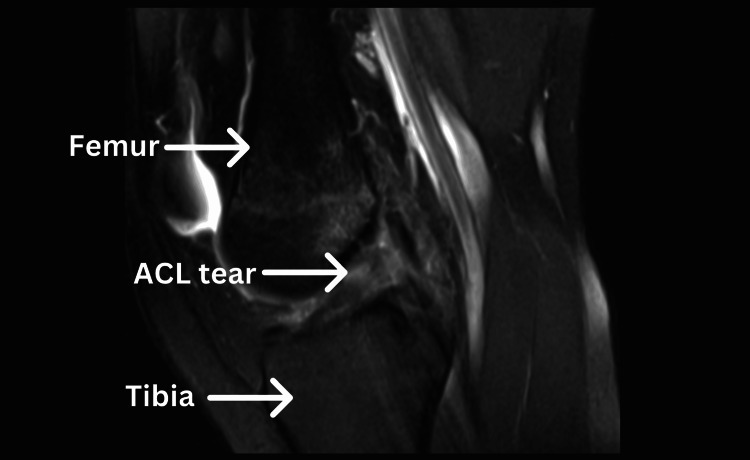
Magnetic resonance imaging (MRI) showing the patient’s torn ACL, along with the labeled femur and tibia ACL: Anterior cruciate ligament.
